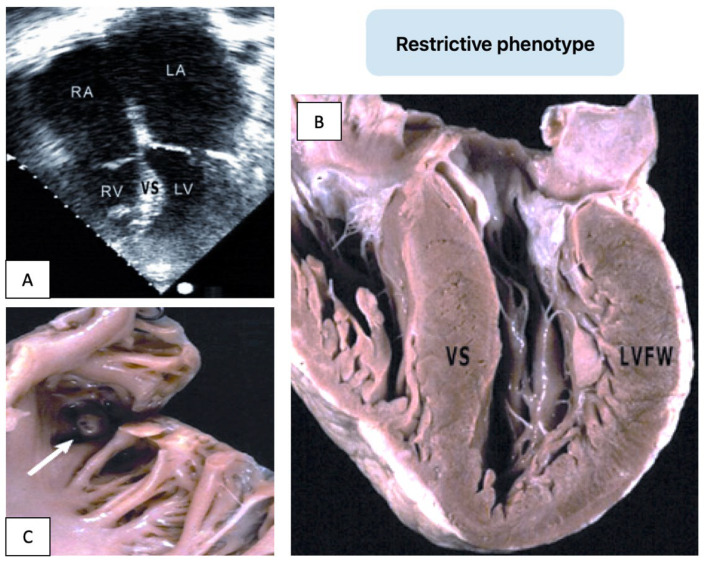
Heart failure in HCM secondary to restrictive physiology and atrial fibrillation. Echocardiographic 4-chamber view (A) and gross pathology findings (B,C) from a male patient with sarcomeric hypertrophic cardiomyopathy (β-myosin heavy chain mutation). Severe dilation of the right (RA) and left atria (LA), normal-sized left (LV) and right ventricles (RV), and mild asymmetric hypertrophy of the ventricular septum (VS) compared to the left ventricular free wall (LVFW) are observed. A thrombus within the LA appendage is indicated by an arrow.
